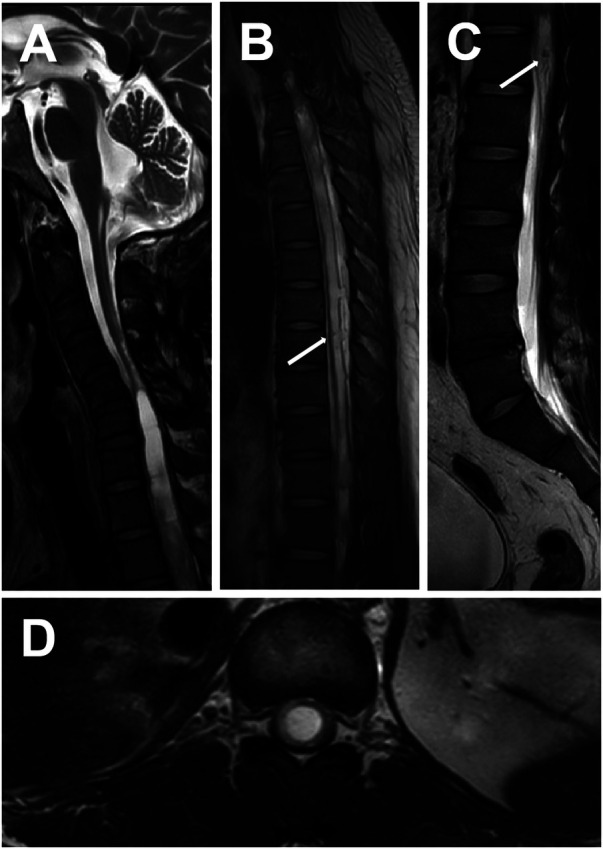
Spinal MRI of Case 1 demonstrating a holocord syrinx involving the entire spinal cord (A–C). Intramedullary hemangioblastomas are indicated by white arrows in (B,C). Axial MRI at the T12–L1 level demonstrating the maximal diameter of the syrinx (D).
